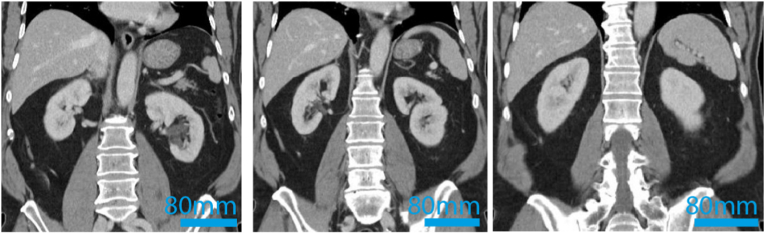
Pre-systemic therapy CT scan of the kidneys ruling out primary tumor of the kidneys.
